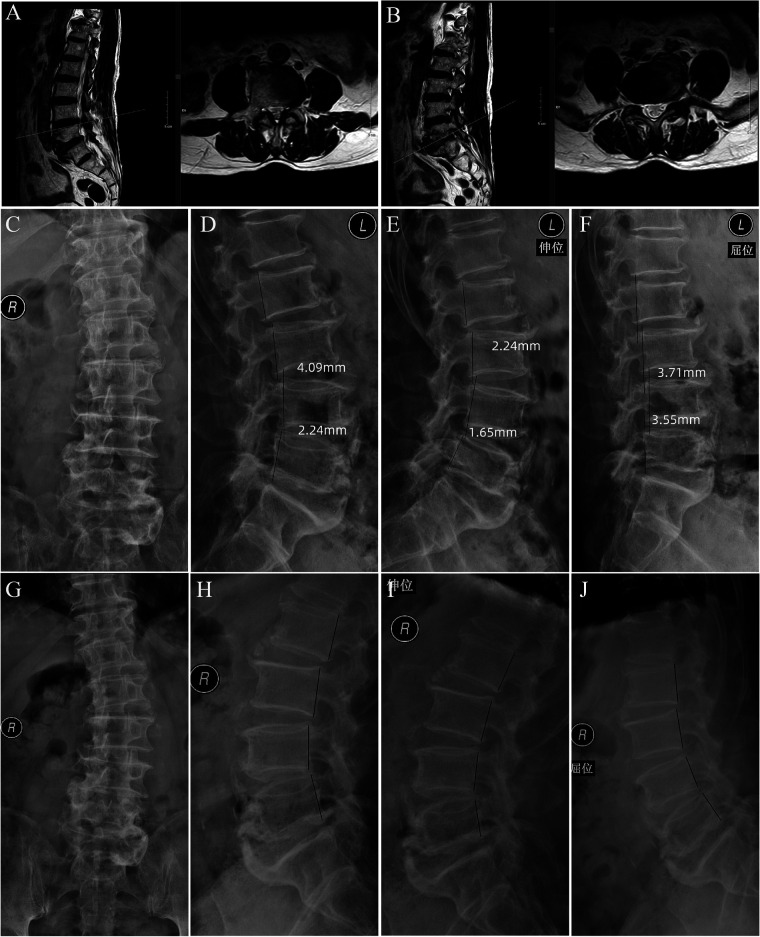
A 72-year-old male patient diagnosed with multilevel lumbar spinal stenosis and grade I spondylolisthesis based on clinical symptoms and imaging findings, treated with the contralateral trans-spinous base inclined (CTI)-AUSS approach. (A) Preoperative L3-4 sagittal and axial MRI images. (B) Preoperative L4-5 sagittal and axial MRI images. (C–F) Preoperative AP, lateral, flexion, and extension radiographs. (G–J) Postoperative AP, lateral, flexion, and extension radiographs. (AP, anteroposterior).
